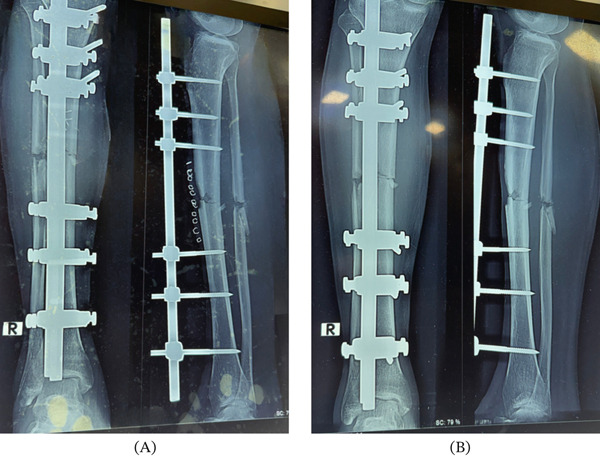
Initial stabilization with external fixation. (A) Anteroposterior and lateral radiographs dated November 29, 2024, showing a right tibial shaft fracture with associated fibular fracture stabilized with a monolateral external fixator after initial wound debridement and primary closure. (B) Anteroposterior and lateral radiographs dated December 27, 2024, showing maintained alignment under external fixation with persistent fracture lines and little callus formation.
